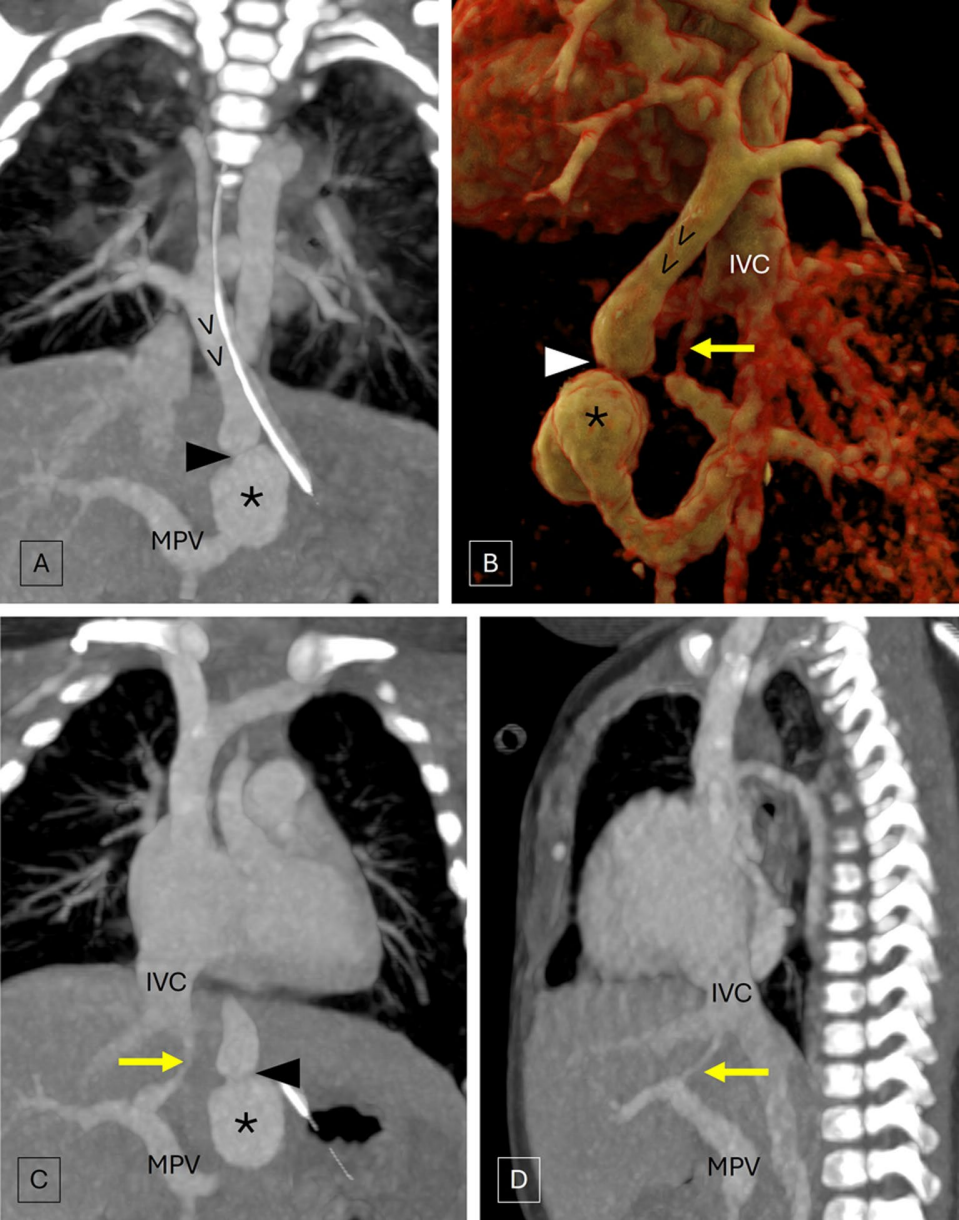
Coronal (A) and volume rendered images (B) of CT angiography depict the union of all four pulmonary veins forming a vertical vein (VV) that descends along the midline and drains into the main portal vein (MPV). Note is made of focal critical stenosis (arrowhead) in the descending channel, just proximal to its confluence with the MPV, with post-stenotic dilatation (*). The coronal (C) and sagittal (D) images demonstrate the patent ductus venosus (yellow arrow) connecting the left branch of the main portal vein and the intra-hepatic inferior vena cava (IVC)
